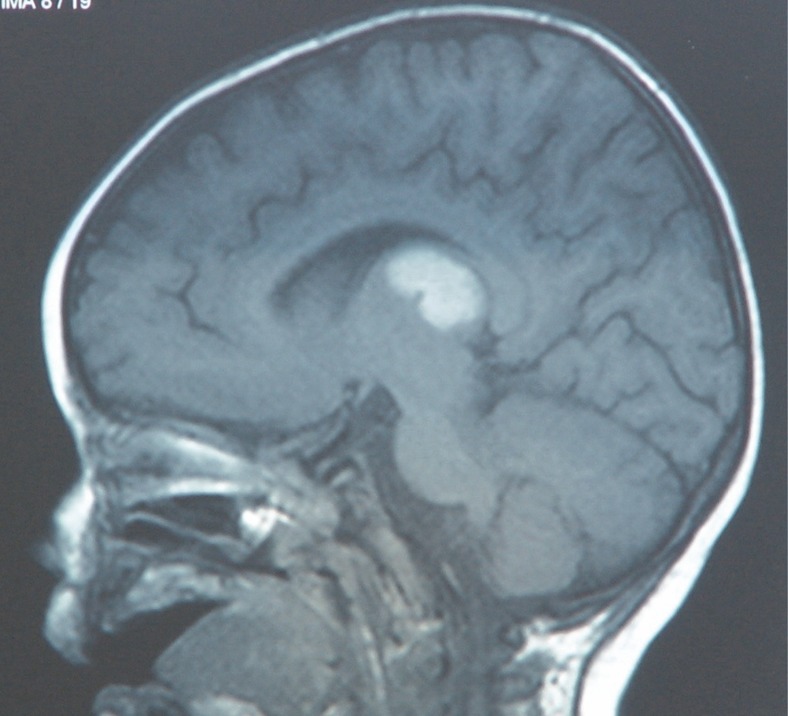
The sagittal view of brain MRI with hyper signal intensity lesion in both thalamus
